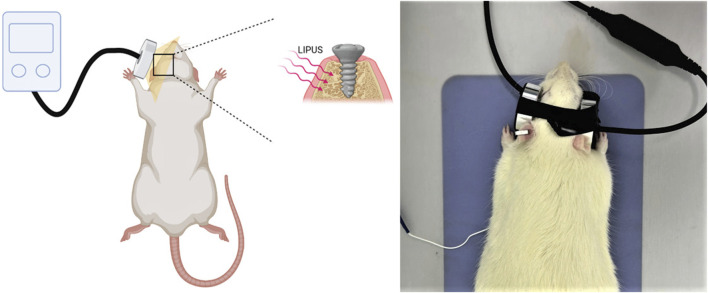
LIPUS treatment. LIPUS, low-intensity pulsed ultrasound.
